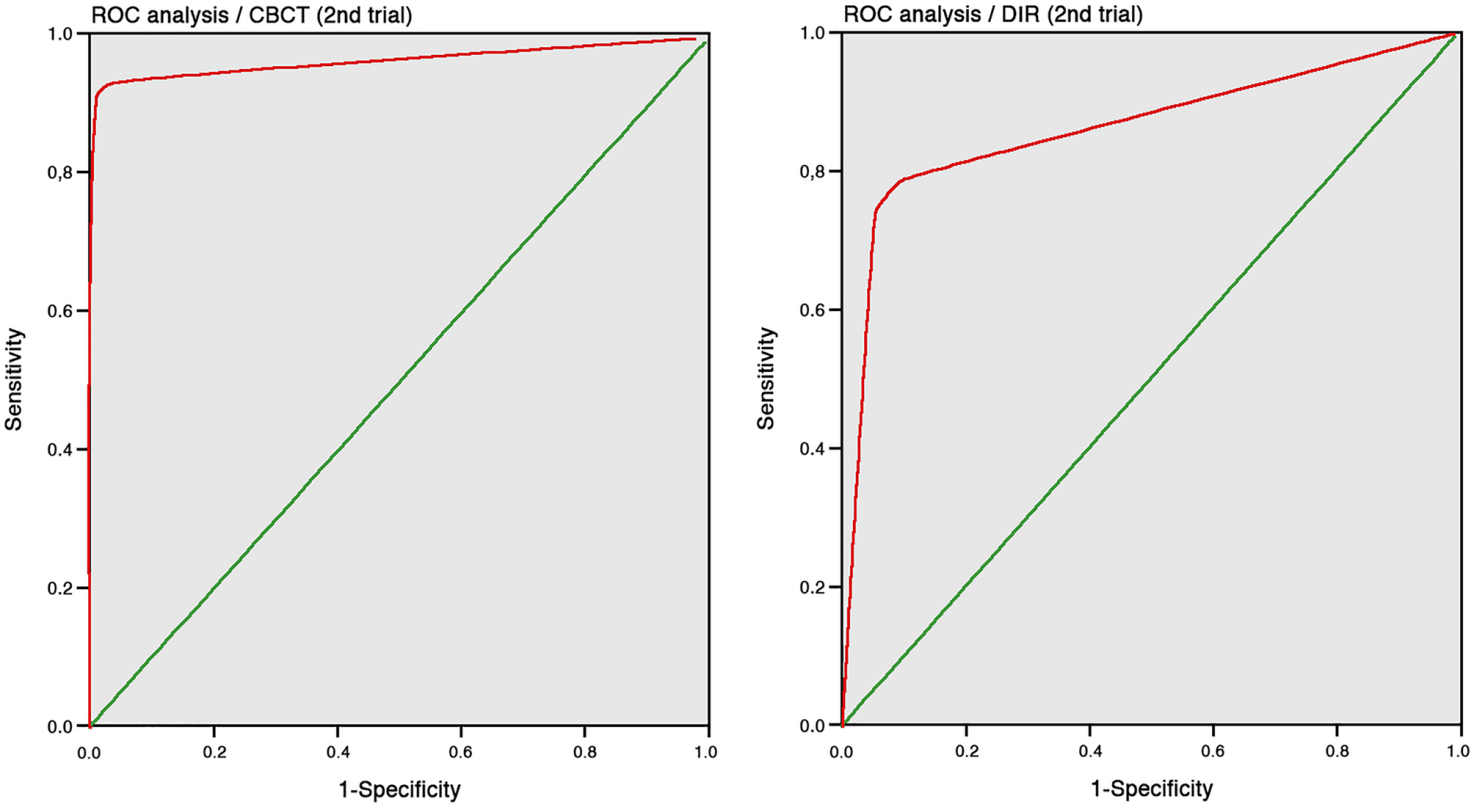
ROC analysis: CBCT (left) and DIR (right) of the second trial being the16 observers pooled with both image acquisition methods.
